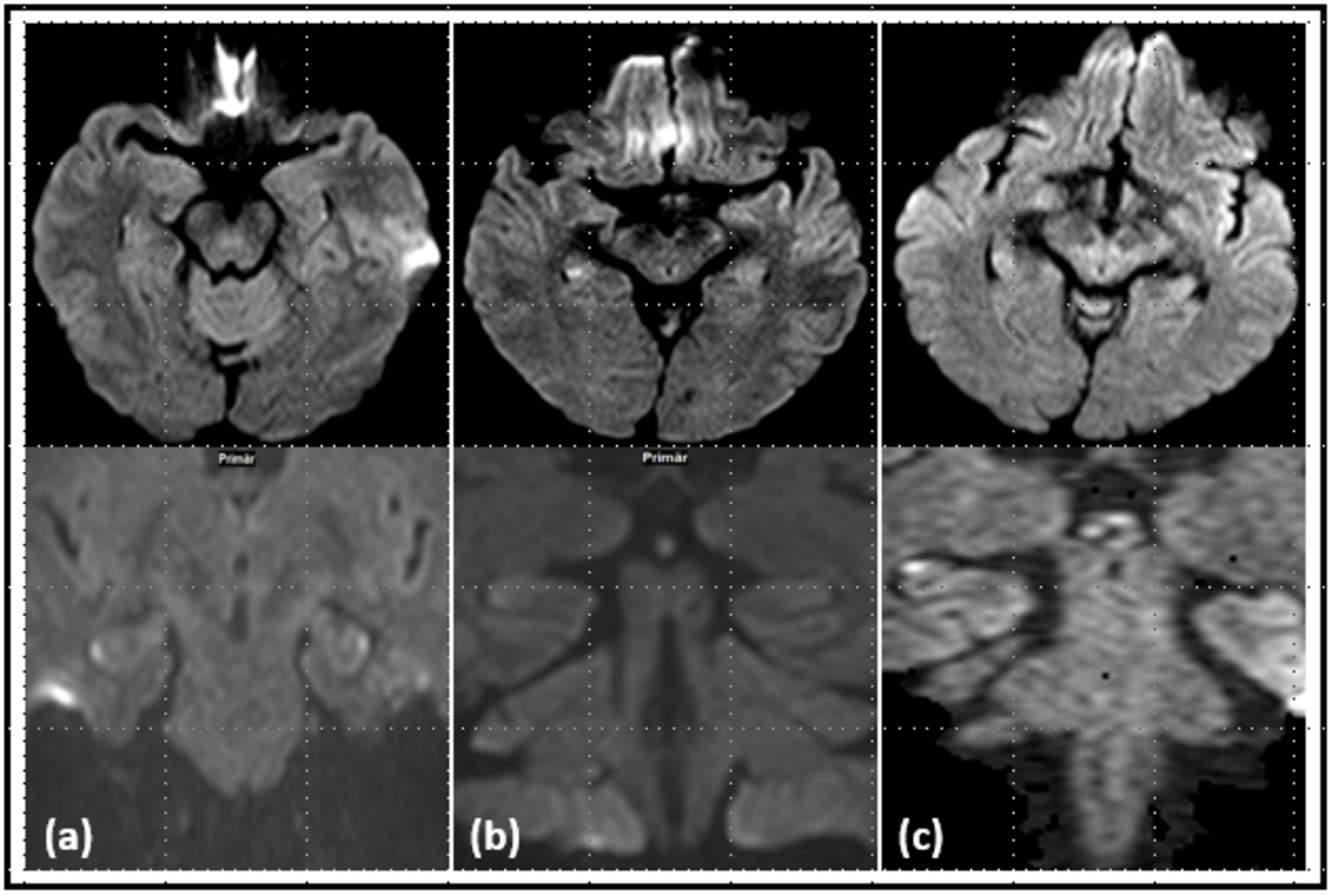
Examples of hippocampal diffusion lesion identified on axial diffusion-weighted imaging (DWI) and coronal DWI (a), hippocampal diffusion lesion more easily identifiable on axial weighted imaging (DWI) compared to coronal DWI (b) and hippocampal diffusion lesion more easily identifiable on coronal DWI (c)
